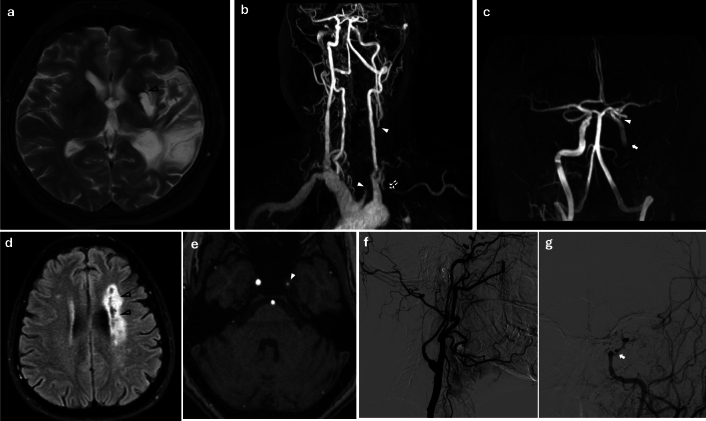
Cranial MRI and MRA scans of Takayasu arteritis patients who presented with stroke. Upper panel shows supra-aortic involvement, while lower panel shows supra-aortic along with medium-sized intracranial arterial vasculitis. (upper panel): A 48-year-old female with a 10-year history of Takayasu arteritis presented with progressive right hemiplegia. a. Axial T2-weighted MRI demonstrates a large hemispheric chronic deep white matter (WM) infarction involving the left capsule-striatal region (black-lined arrowhead) as well as the posterior temporal lobe and angular gyrus. b. Contrast-enhanced MR angiography displayed the chronic occlusion of the left common carotid artery (CCA), extending approximately 1 cm from its origin at the aortic arch to the bifurcation level (white arrowheads). The non-enhancing segment between the two arrowheads indicates the extent of the long-segment CCA occlusion. The chronic occlusion of the left subclavian artery proximal segment is also visualized (dashed arrow). c. Time-of-Flight (TOF) MR angiography without contrast shows a normal-appearing intracranial segment of the left internal carotid artery (ICA) (arrowhead). However, no flow signal is detected in the distal cervical and petrous segments of the left ICA (downstream of the white arrow), consistent with retrograde flow originating from the contralateral, normal side through the anterior communicating artery. (lower panel): The 57-year-old female patient, previously known as Takayasu arteritis, presented with right hemiparesis with paraphasia. d. axial FLAIR image shows a wide zone of leukomalacia with central rarefaction involving the supraventricular WM and corona radiata, consistent with large deep WM infarcts reflecting the water-shed zones (black-lined arrowheads). e. TOF MR angiography depicted marked concentric intima-media thickening with hemodynamically significant narrowing in the cavernous segment of the left ICA (white arrowhead), representing another focus of vasculitic involvement. f. Subsequent Digital Subtraction Angiography (DSA) from the left CCA injection displayed severe narrowing and contour abnormalities along the entire cavernous-supra-clinoid segment of ICA (black arrows), suggesting vasculitic involvement. Enlarged branches of the left external carotid artery, such as the internal maxillary artery and the prominent left middle meningeal artery, reconstitute the salvage collateralizations beyond the stenotic left ICA segment. g. Further DSA with selective catheterization of the left ICA showed steno-occlusive narrowing of the left cavernous ICA after removing the collateral contributions effect (white arrow)
